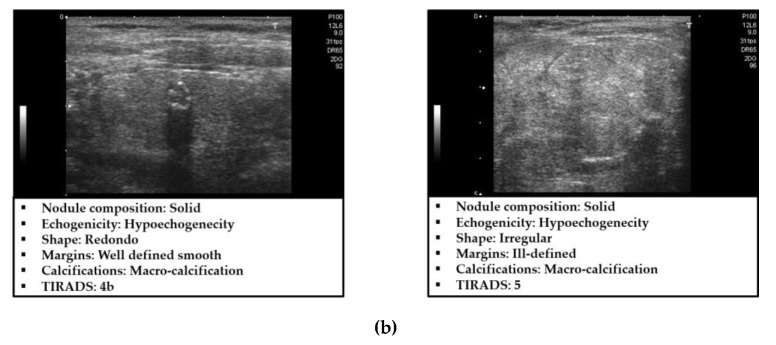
Example of captured ultrasound thyroid images in the thyroid digital image database (TDID) dataset [20]: (a) benign cases and (b) malign cases.
